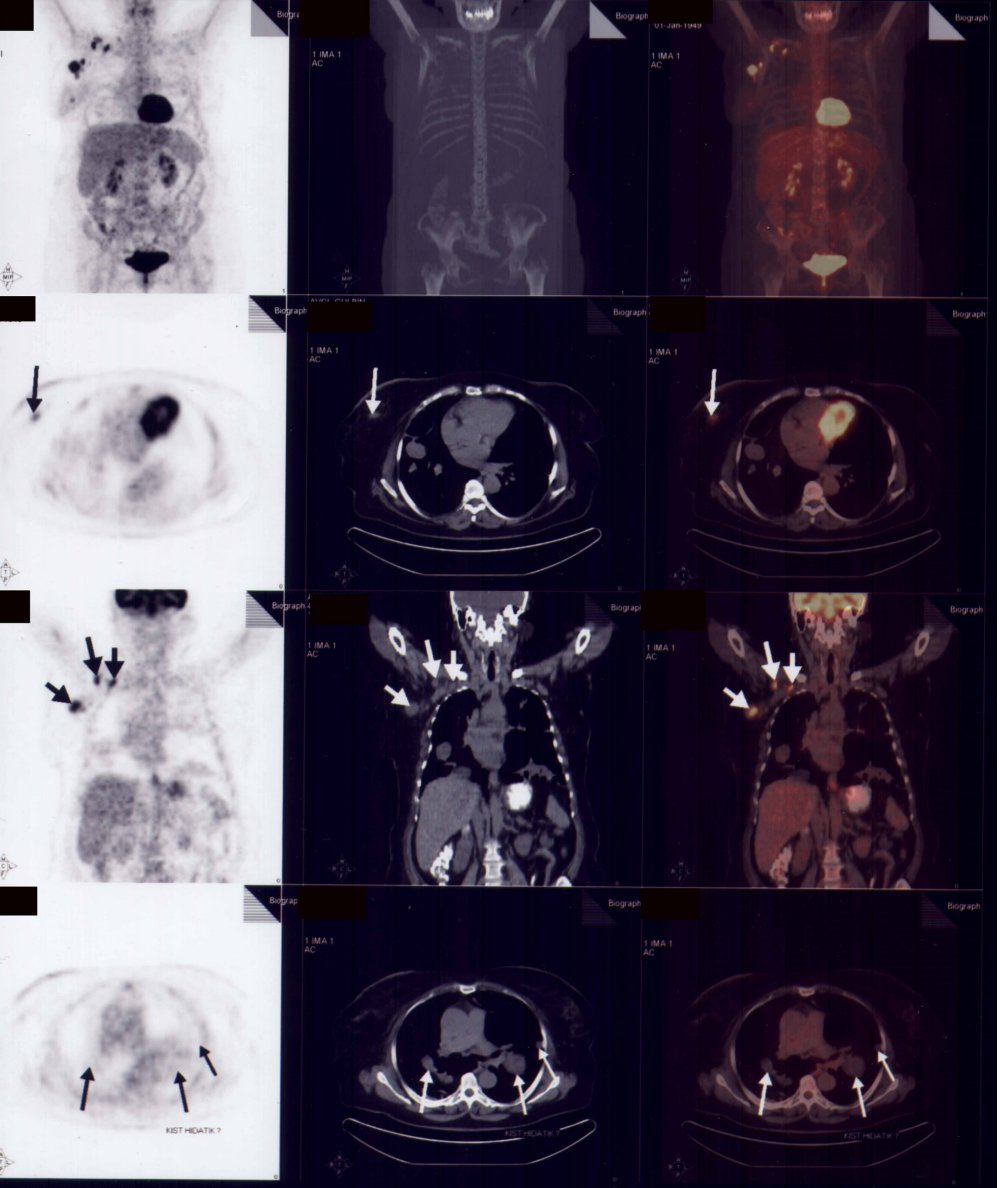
FDG PET scans, First Column of the Figure showed increased FDG uptake in both breast cancer and in metastatic axillary and supraclavicular lymph nodes (arrows) and also no FDG Uptake of the benign pulmonary lesions (small arrows).
